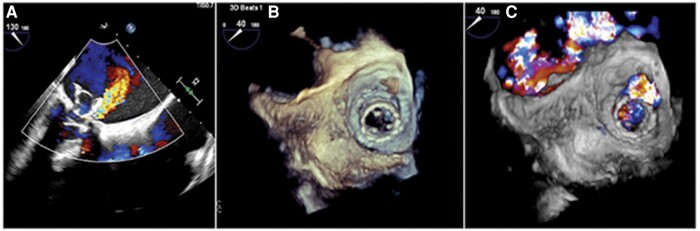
Sequence of pre-procedural echocardiogram images showing displacement of the prosthetic mitral valve towards the left atrium with a significant periprosthetic regurgitation. (*A*) Transthoracic echocardiogram Doppler colour image. (*B* and *C*) 3D echocardiogram [51.0 × 159 mm (×DPI)].
